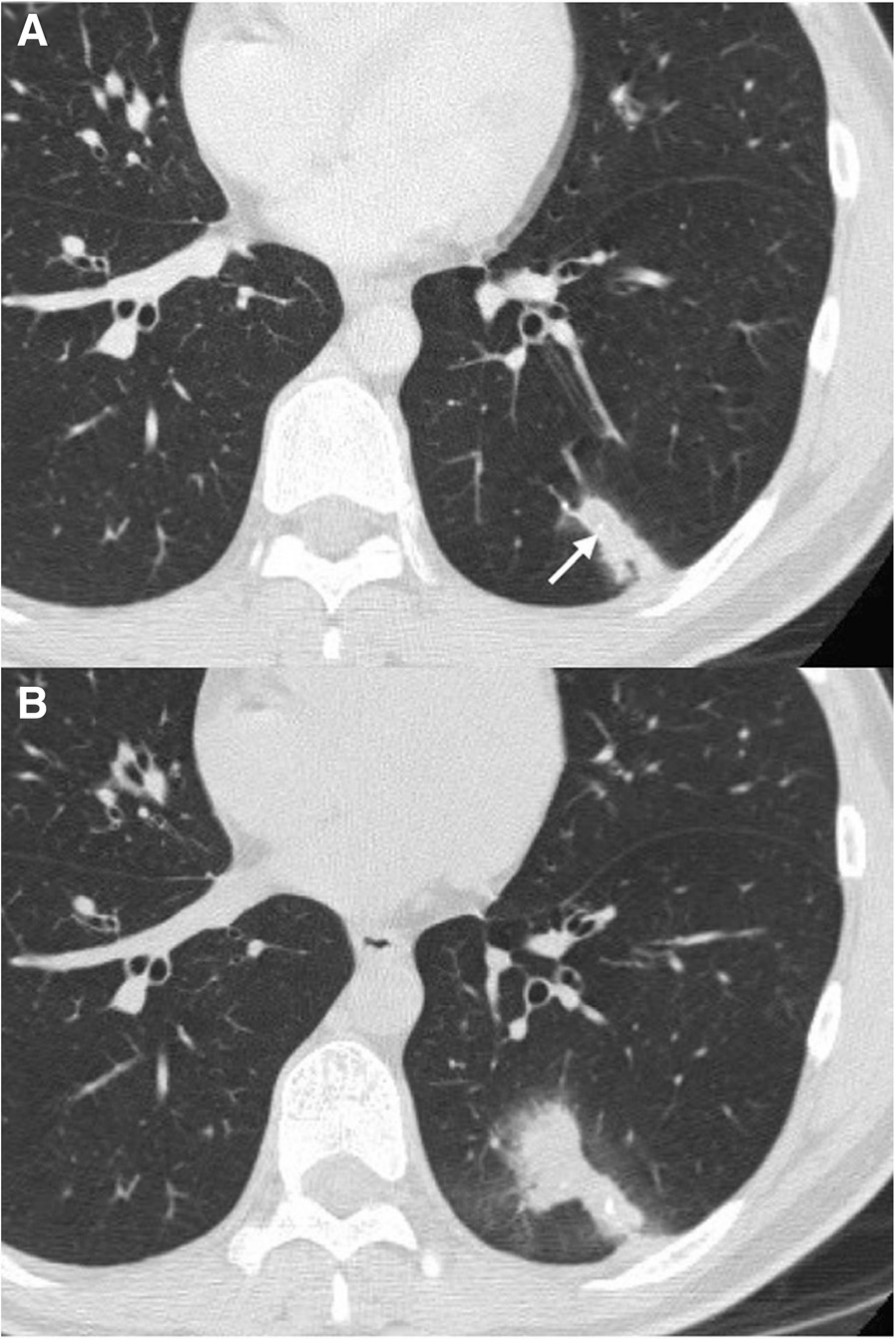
Computed tomography scan of the chest. a Chest computed tomography showed an irregularly shaped solitary nodule in the periphery of the left lower lobe, with microcalcifications (arrow) and pleural indentation. b After 2 weeks, the nodules became enlarged, around which ground glass opacity appeared
